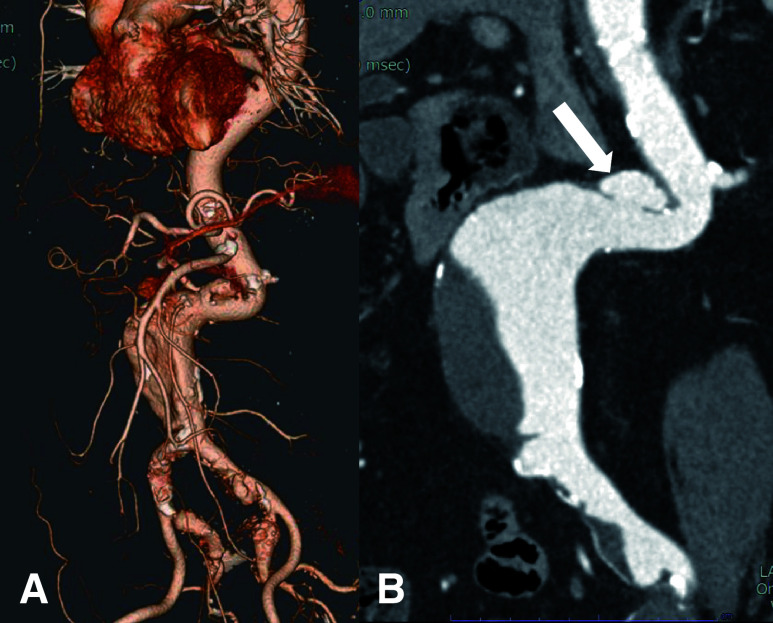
Fig. 1 Preoperative 3-dimensional reconstruction and CTA. (A) 3-dimensional reconstruction confirmed infrarenal AAA with severely angulated neck. Aneurysmal dilatation of left common iliac artery and atherosclerotic atheroma of bilateral iliac arteries were observed. (B) Preoperative CTA showed the infrarenal AAA with a saccular aneurysm (white arrow) due to a PAU in the proximal landing zone. CTA: computed tomography angiography; AAA: abdominal aortic aneurysm; PAU: penetrating atherosclerotic ulcer.
